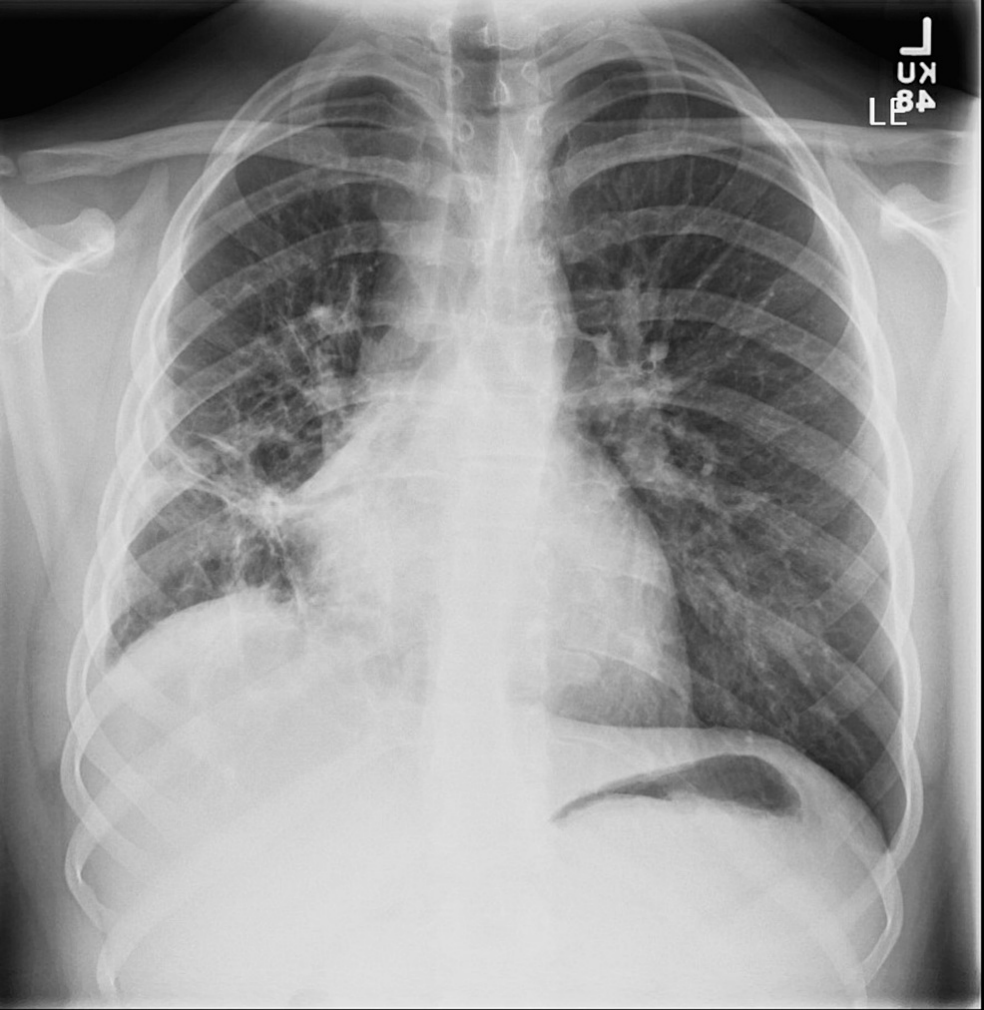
Chest X-rays after four months of treatment Interval improvement of right lower lobe patchy opacity with residual atelectasis in the right middle and lower lobes is observed. There was right pleural thickening, but no effusion was seen.
